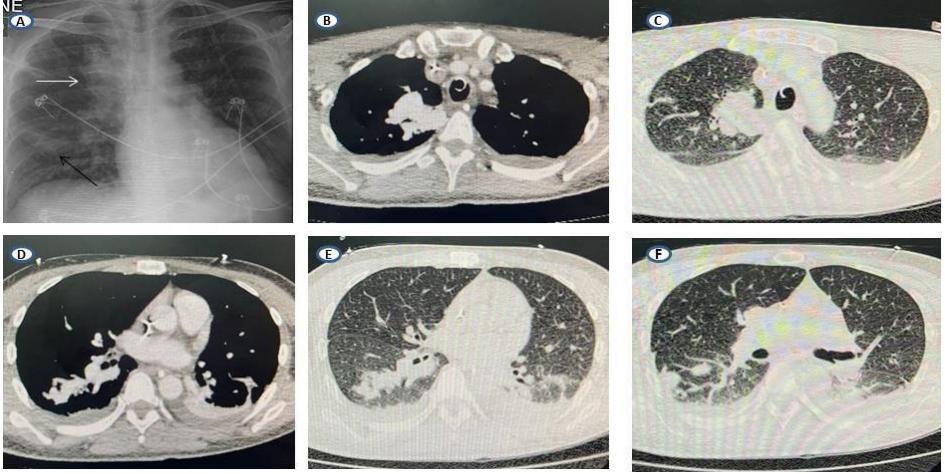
Pulmonary radiological examination. In A) chest X-ray showing arteriovenous malformation (AVM) in the apical segment of the upper right lobe (URL) – white arrow – and the apical segment of the right lower lobe (RLL) – black arrow; in B and C) computed tomography (CT) of the chest with contrast showing AVM in the URL; and in D, E, and F) chest CT with contrast showing AVM in the RLL and the apical segment of the left lower lobe.
